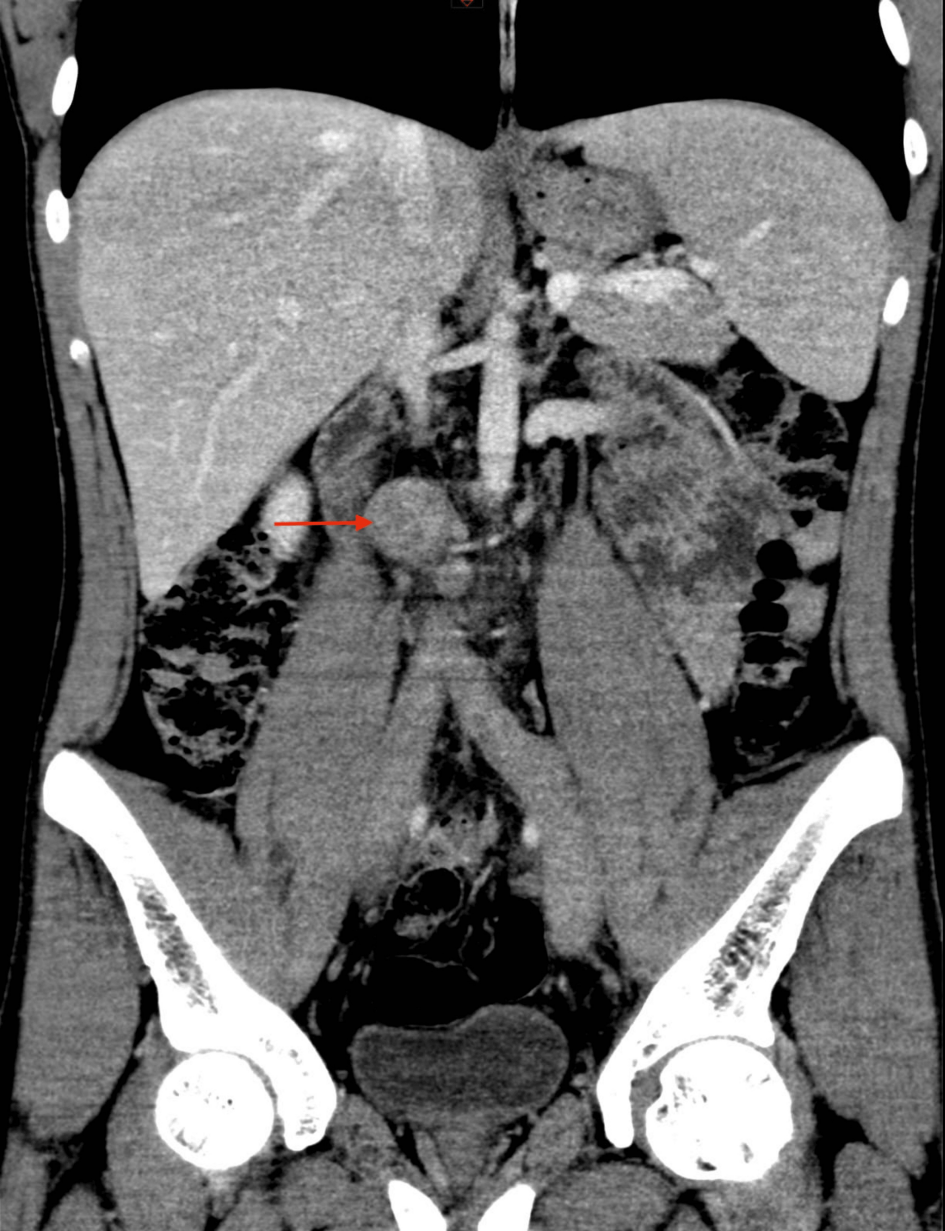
CT coronal image of the abdomen showing ectatic intrabdominal IVC with non-opacified lumen showing complete thrombus (red arrow) IVC: inferior vena cava
